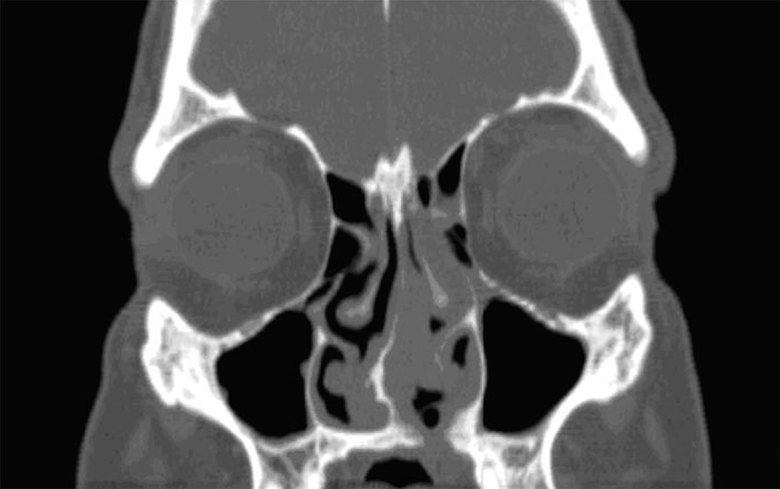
Patient 2. Coronal CT scan showing a nasal mass measuring 2.6 × 3.1 × 2.9 cm predominantly to the left of the nasal septum and extending across the midline to the right. The lesion also extends into the premaxillary space. The erosion through the left hard palate is significant.
